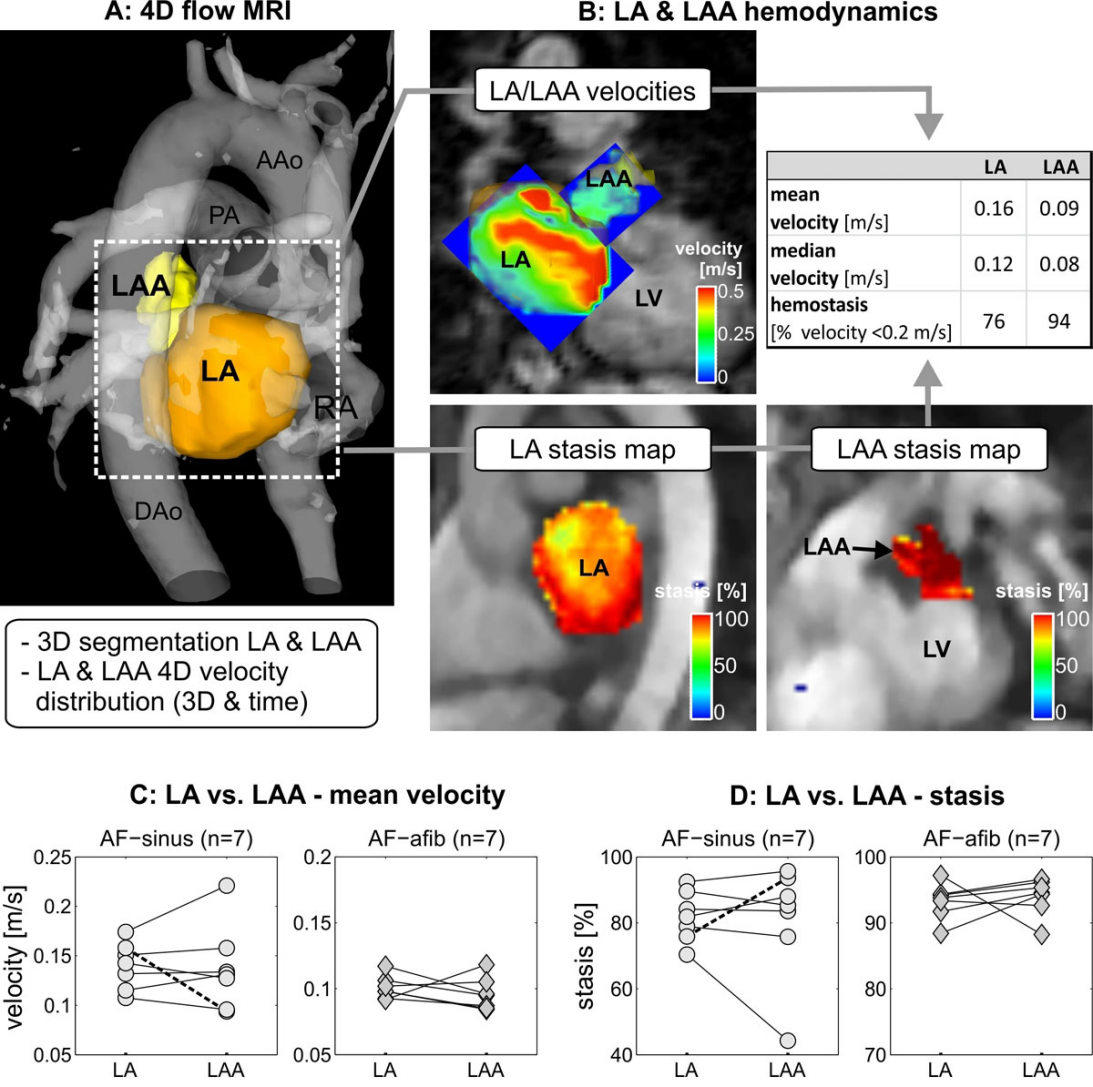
4D flow MRI (A) including 3D segmentation of the LA and LAA and calculation of LA and LAA stasis maps (B). Note the increased stasis in the LAA vs. LA in this AF patient. C, D: Mean velocity and relative stasis in the LA compared to the LAA in n14 AF patients. Dashed line = patient example in A,B.
